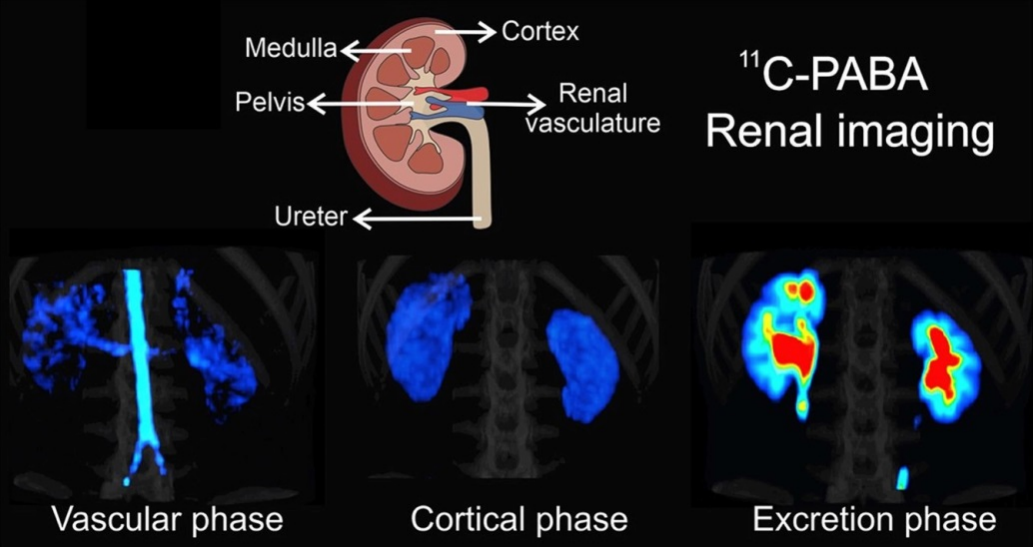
^ 11^C-para-Aminobenzoic acid (^11^C-PABA) PET/CT renal imaging in a healthy subject. After a vascular phase after 22 seconds after injecvtion, the cortical uptake was evident within 2.7 and 3.2 minutes, with maximal parenchymal uptake in the renal cortex. The excretory phase was seen after 9 minutes indicating the maximal uptake in the pelvicalyceal system. Modified from Ruiz-Bedoya et al 12, copyright 2020 by the Society of Nuclear Medicine and Molecular Imaging, Inc.
